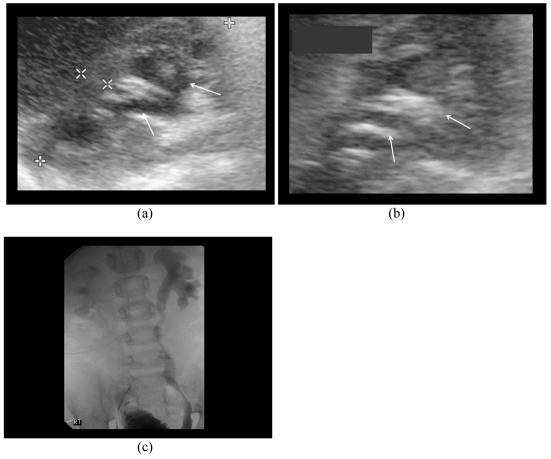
A seven years-old boy had spina bifida and neurogenic bladder. a) The sonogram shows left mild to moderate hydronephrosis (arrows) with thinning of renal cortex; b) ECS demonstrates presence of microbubbles in the dilated PCS and increased dilatation of the PCS (arrows); c) MCU shows left Grade IV VUR.
